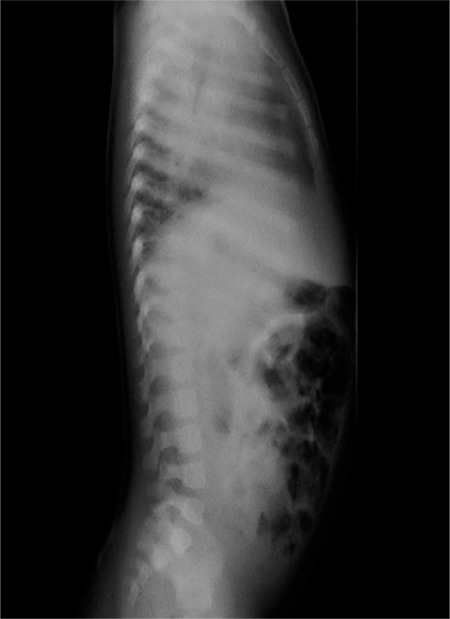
Radiography scan revealed mild reduction in thoracic vertebrae corpus height, with irregularities in upper and lower plateau as well as lordosis
